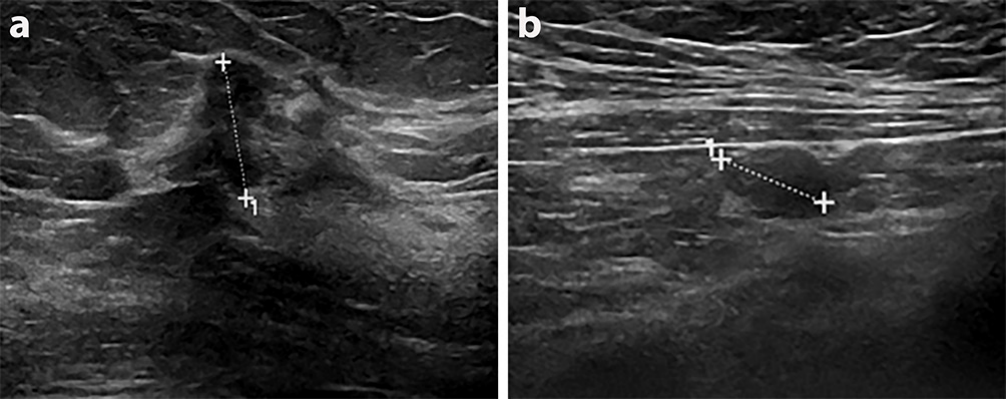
Corresponding sonographic image shows an irregular hypoechoic 2 cm likely malignant mass (a) with likely pathological right axillary lymph node (b).
